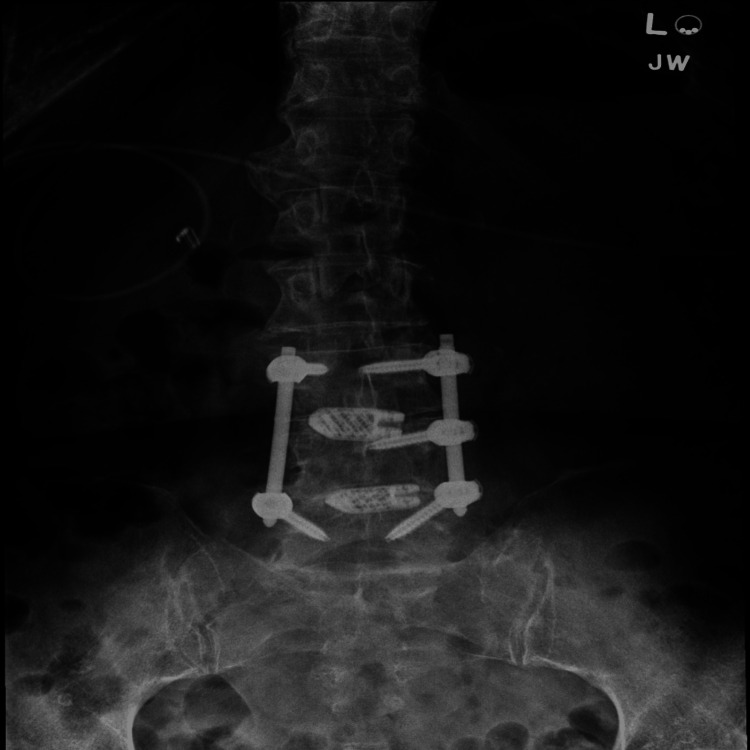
An X-ray of the lumbar spine (anteroposterior view); left transforaminal lumbar fusion L3-L5 is visualized
